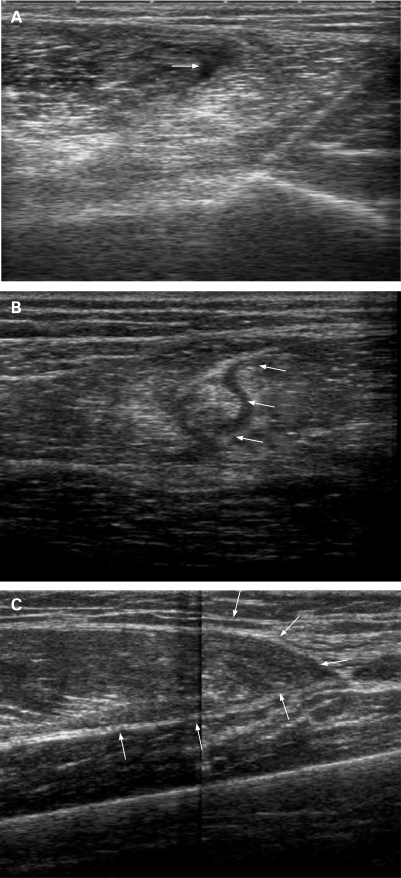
Ultrasonographic appearance of different grades of muscle lesion: (A) grade I lesion; (B) grade II lesion; (C) grade III lesion.
